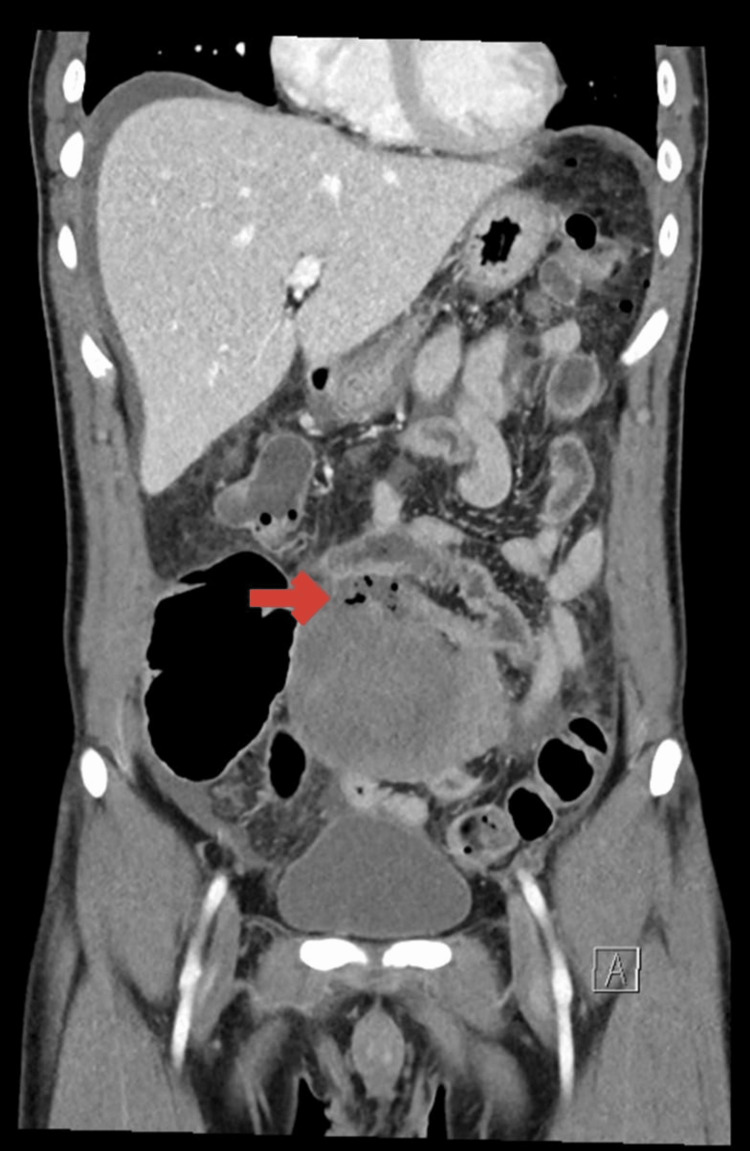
CT abdomen coronal view showing the presence of a large mass with the presence of multiple air foci CT, computed tomography
